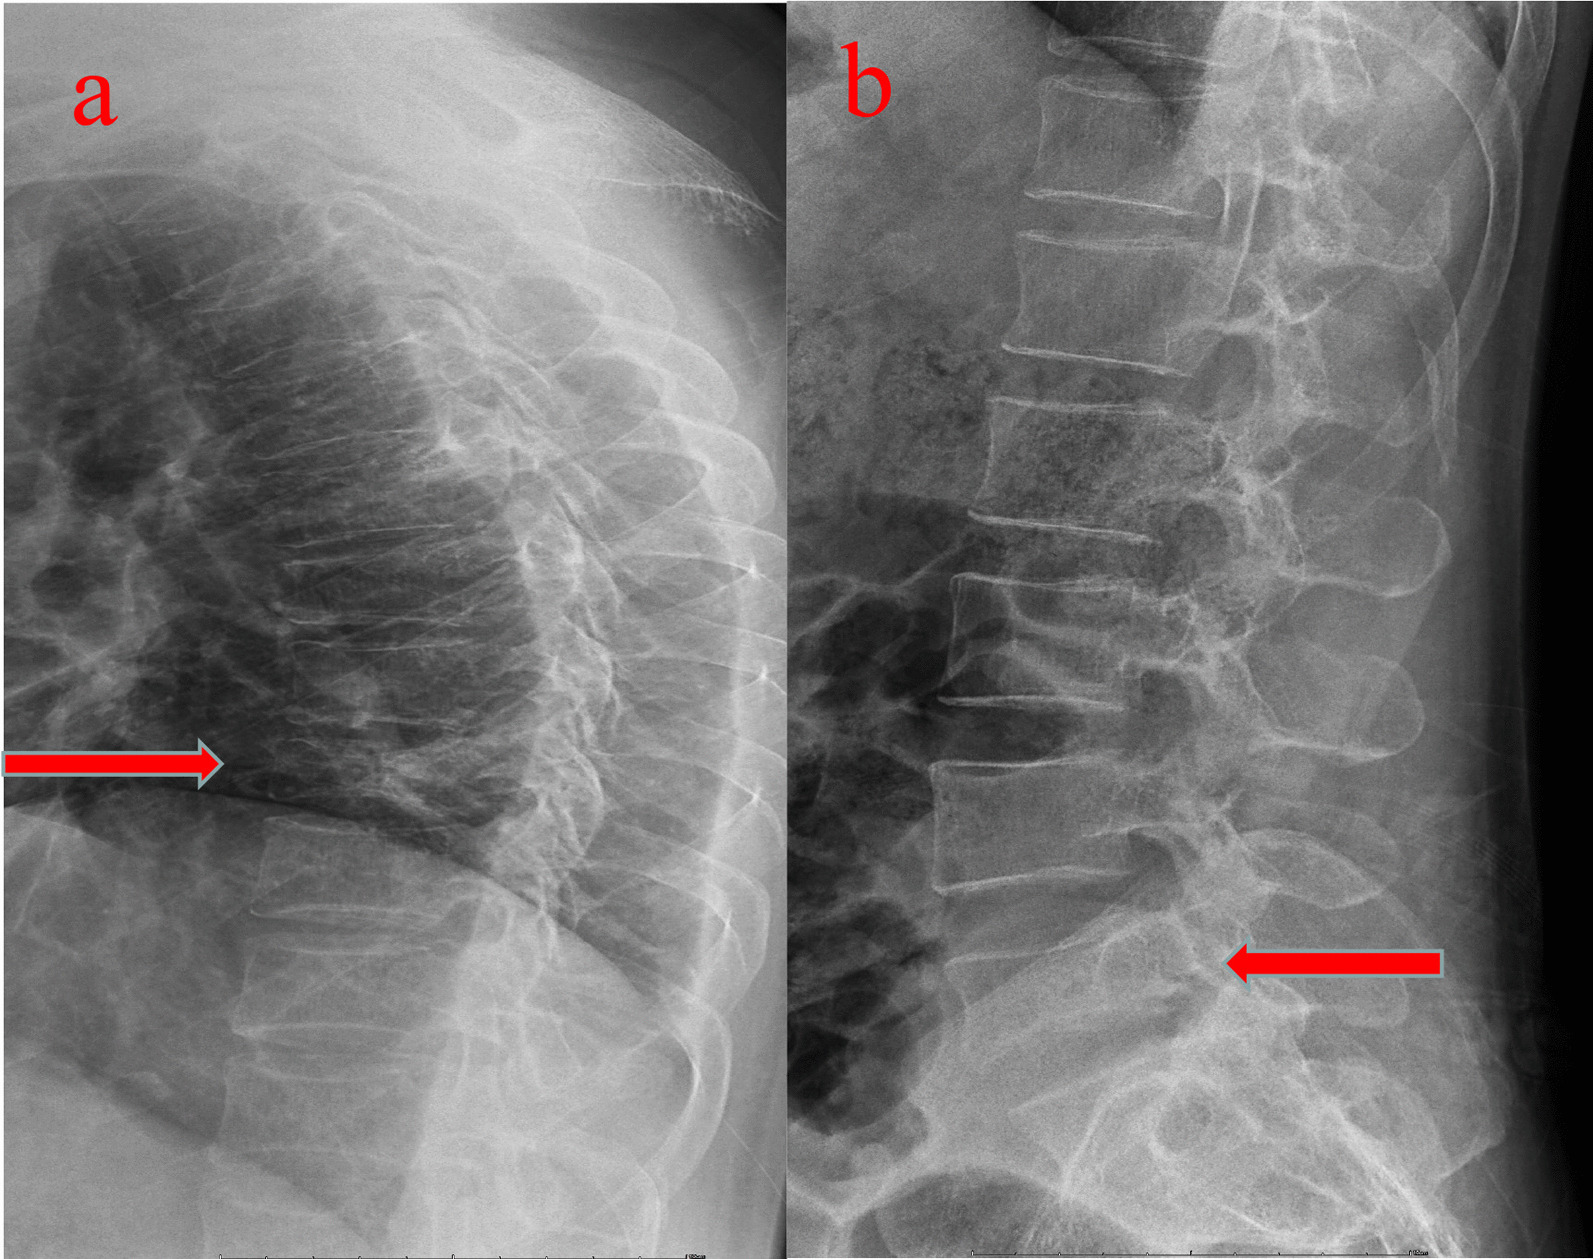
Radiology date of the patient at the time of injury. a The lateral X-ray showed 9th thoracic (T9) vertebral compression fractures (VCFs). b The lateral X-ray showed 5th lumbar (L5) VCFs. Arrows showed the T9 and L5 fracture
